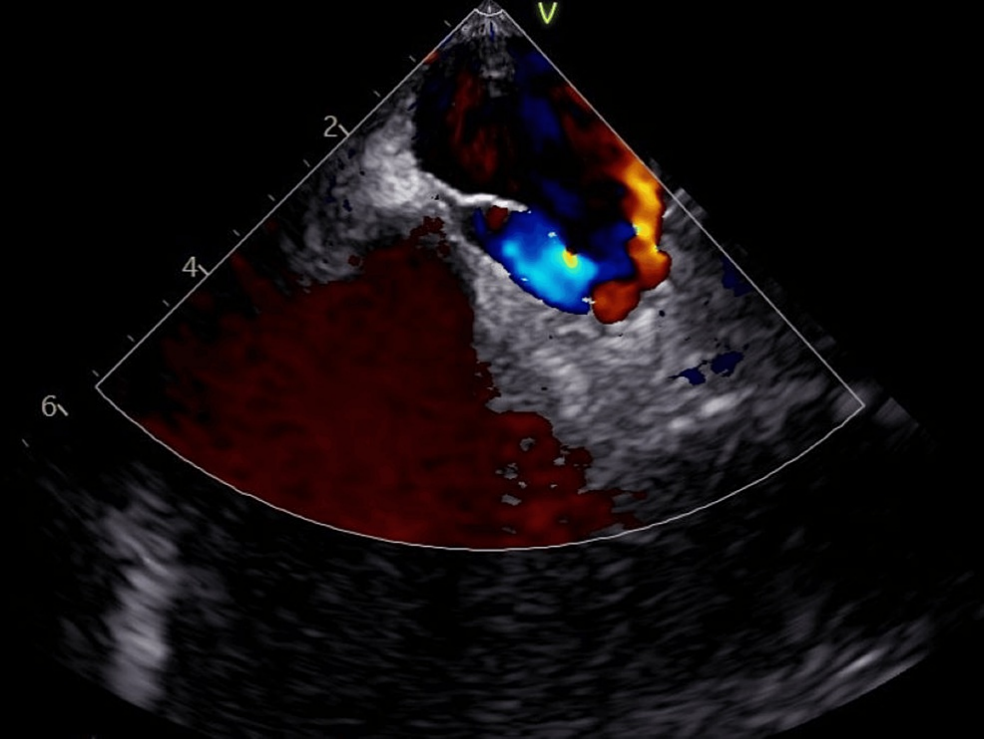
PFO with right-left shunt in transesophageal echocardiogram with color Doppler PFO: patent foramen ovale
